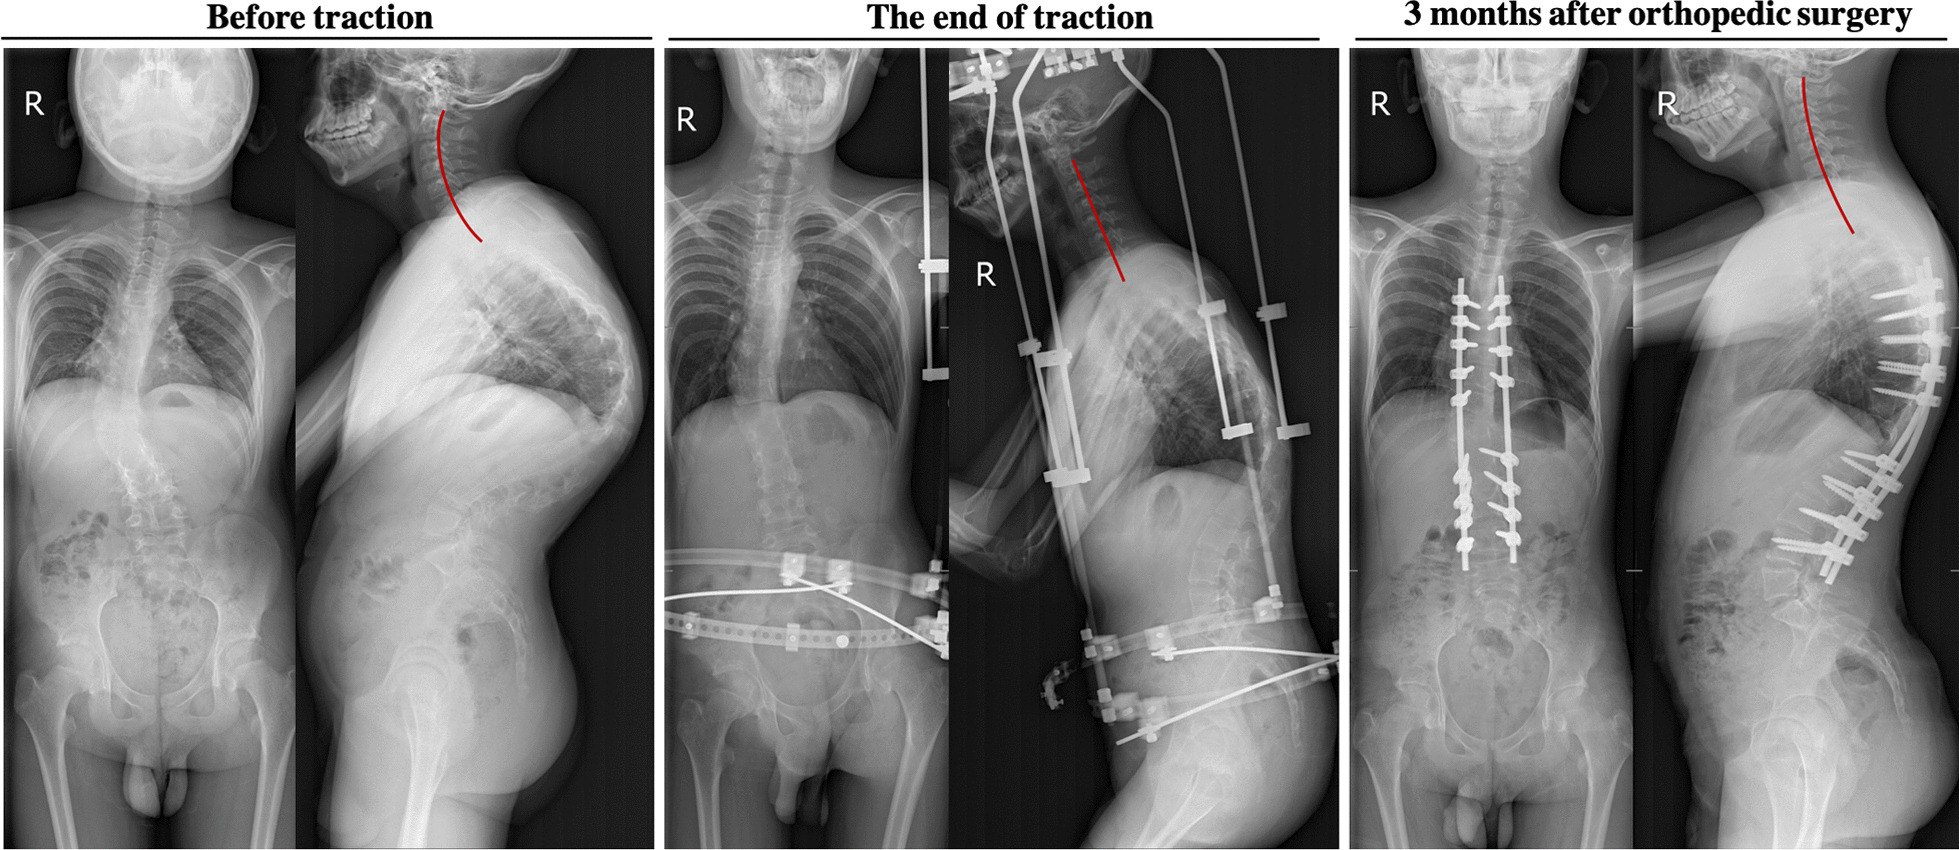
A 13-year-old male, with a height of 136 cm and a weight of 40 kg, was diagnosed with severe rigid spinal deformity. The operation time was 31 min, intraoperative blood loss was 5 ml, and the total traction duration was 60 days. After traction, the height was 138 cm and the total traction volume was 12 cm. X-ray examination showed that the cervical curvature was significantly straightened after Halo-pelvic fixation brace traction, and the cervical spine sagittal alignment was significantly restored after removal of the fixation brace
